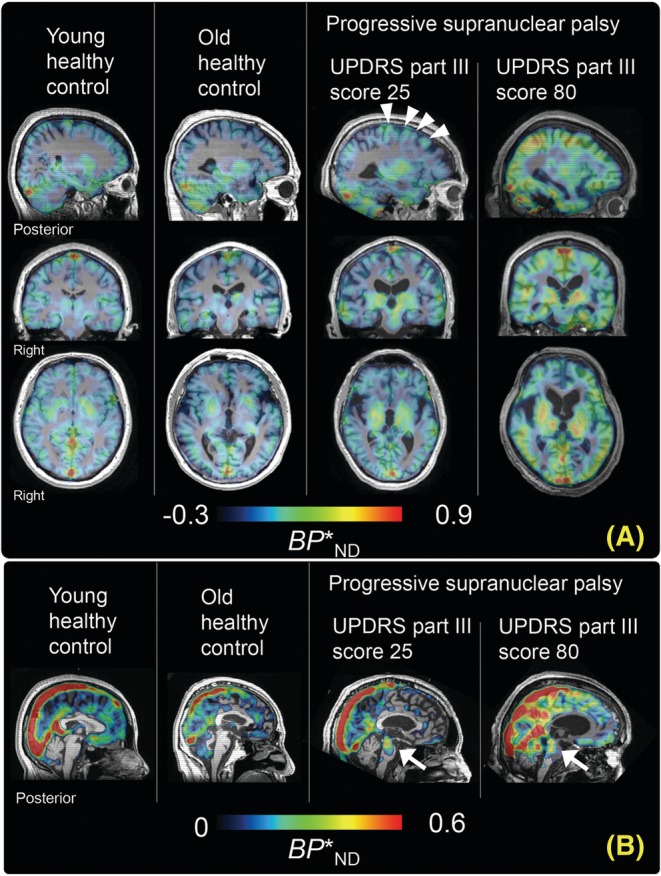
Representative parametric images of [^11^C]PBB3 *BP_ND_ in 1 young HC (age, 35 years) and 1 old (age, 75 years) HC and 2 patients with PSP with mild (age, 57 years; UPDRS, 25 points) and severe (age, 75 years; UPDRS, 80 points) symptoms (focusing on neocortex [A] and brainstem [B]). PET data are fused on corresponding T_1_‐weighted MR images. Arrows indicate increased radioligand binding in the midline area of the PSP midbrain. Arrow heads show increased radioligand binding in the lateral area of the PSP frontal lobe. Please note that there is a difference in dynamic range between (A) and (B) because values of [^11^C]PBB3 *BP_ND_ in white matter including brain stem are smaller than those in gray matter. Binding potential (*BP
